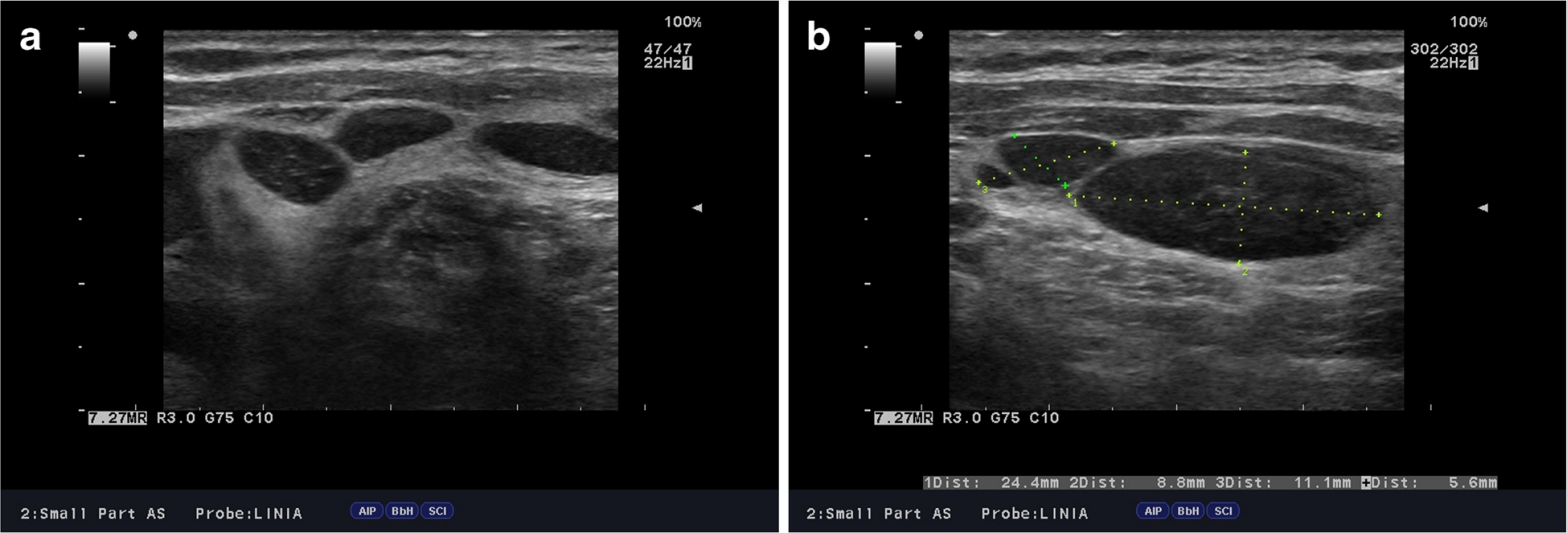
(a,b) Ultrasound image of cervical lymph node packages located along the sternocleidomastoid muscle in 57-year-old female with SLL-B/CLL-B.
